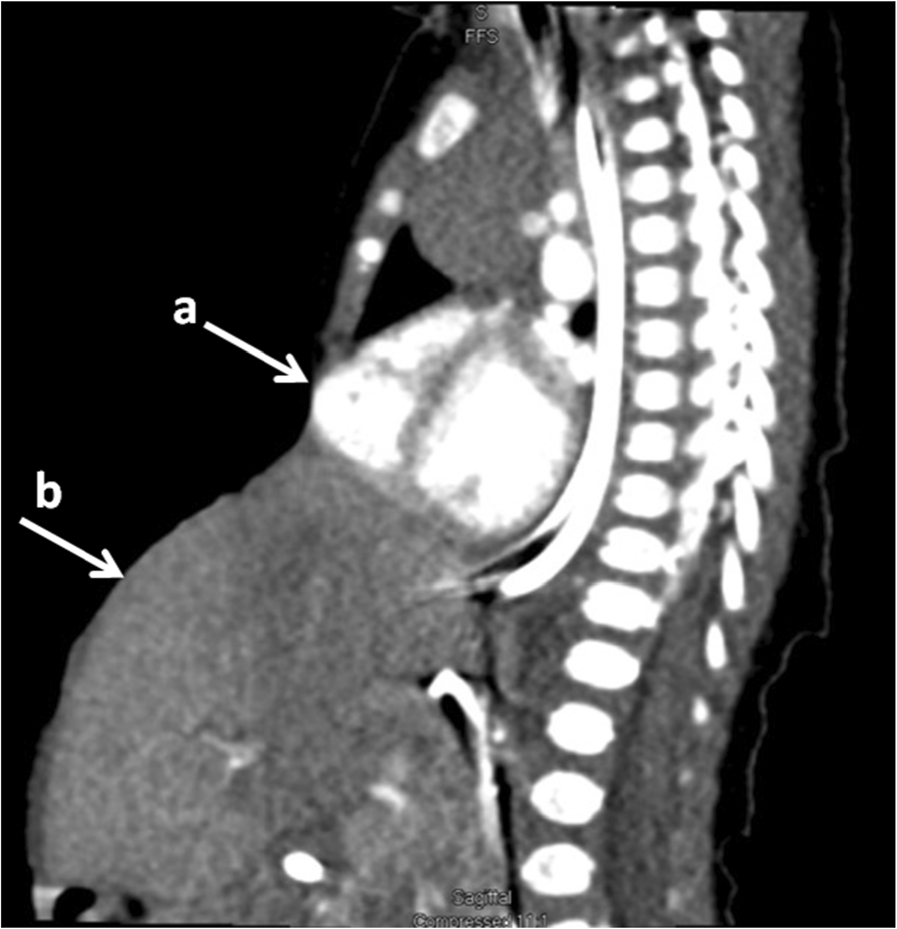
Computed tomography scan. A sagittal view from a contrast-enhanced computed tomography study demonstrating protrusion of the right ventricle through the sternal defect (a) and extra-abdominal position of the organs in the large omphalocele (b).
